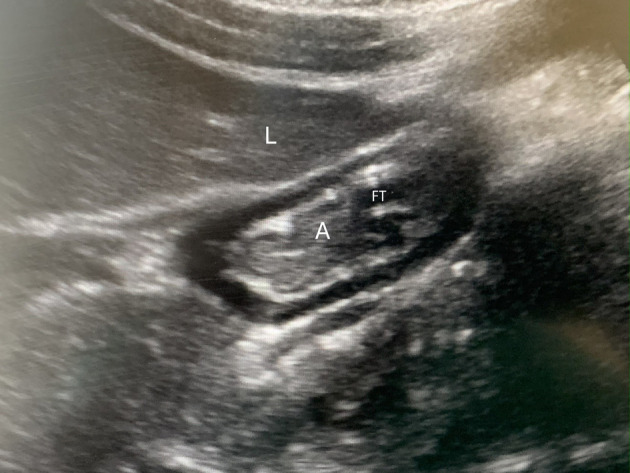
Gastric ultrasound in a patient receiving post-pyloric feedings via feeing tube (FT) showing the collapsed antrum (A) with the classic “bull’s eye appearance,” positioned under the liver (L).
